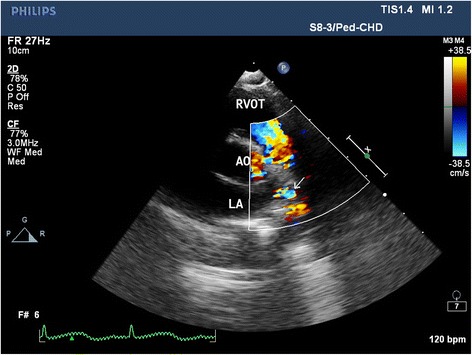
Postoperative echocardiograph demonstrating the blood flow of the aorta and left superior pulmonary vein (LA = left atrium; AO = aorta; RVOT = right ventricular outflow tract; *white arrow* = the left superior pulmonary vein draining into the left atrial appendage)
